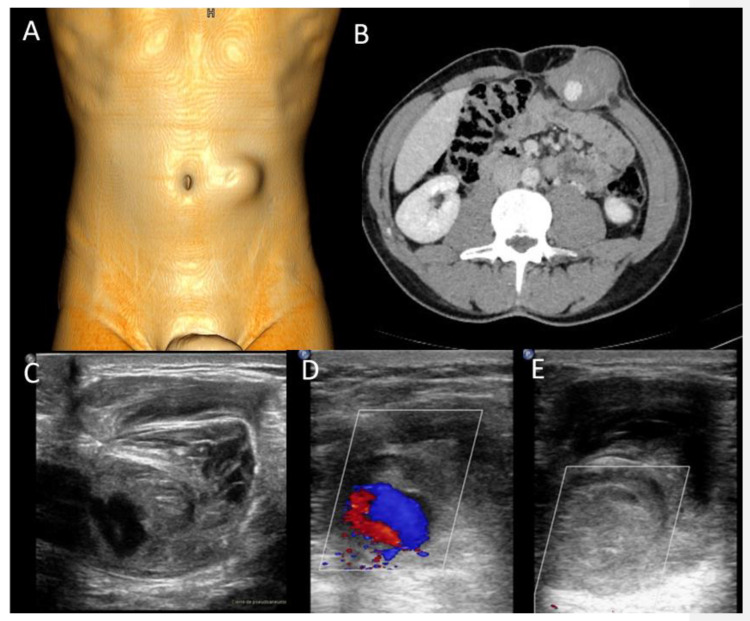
A 37-year-old man presenting with a pulsatile mass with trauma history. Volume rendering cutaneous reconstruction (A) and axial, contrast-enhanced portal-phase CT (B) showed the presence of a large post-traumatic pseudoaneurysm of the inferior right epigastric artery, with thrombotic apposition. B-mode US and CDUS (C–E) demonstrate the presence of an anechoic part, corresponding to the contrast-enhanced part on CT, and the heterogeneity of the thrombus. CDUS (D,E) shows the “yin–yang sign”. The pseudoaneurysm was promptly treated with thrombin injection.
